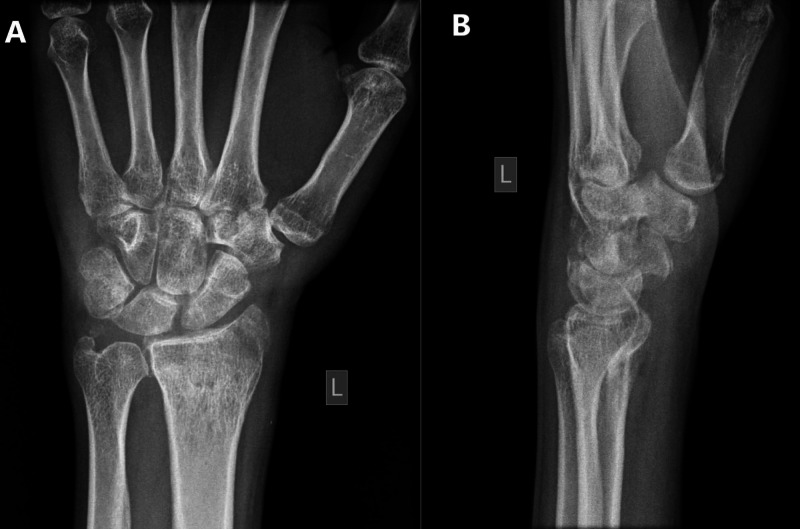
X-ray of the left wrist before discharge. A) Anteroposterior (AP) view; B) Lateral view
